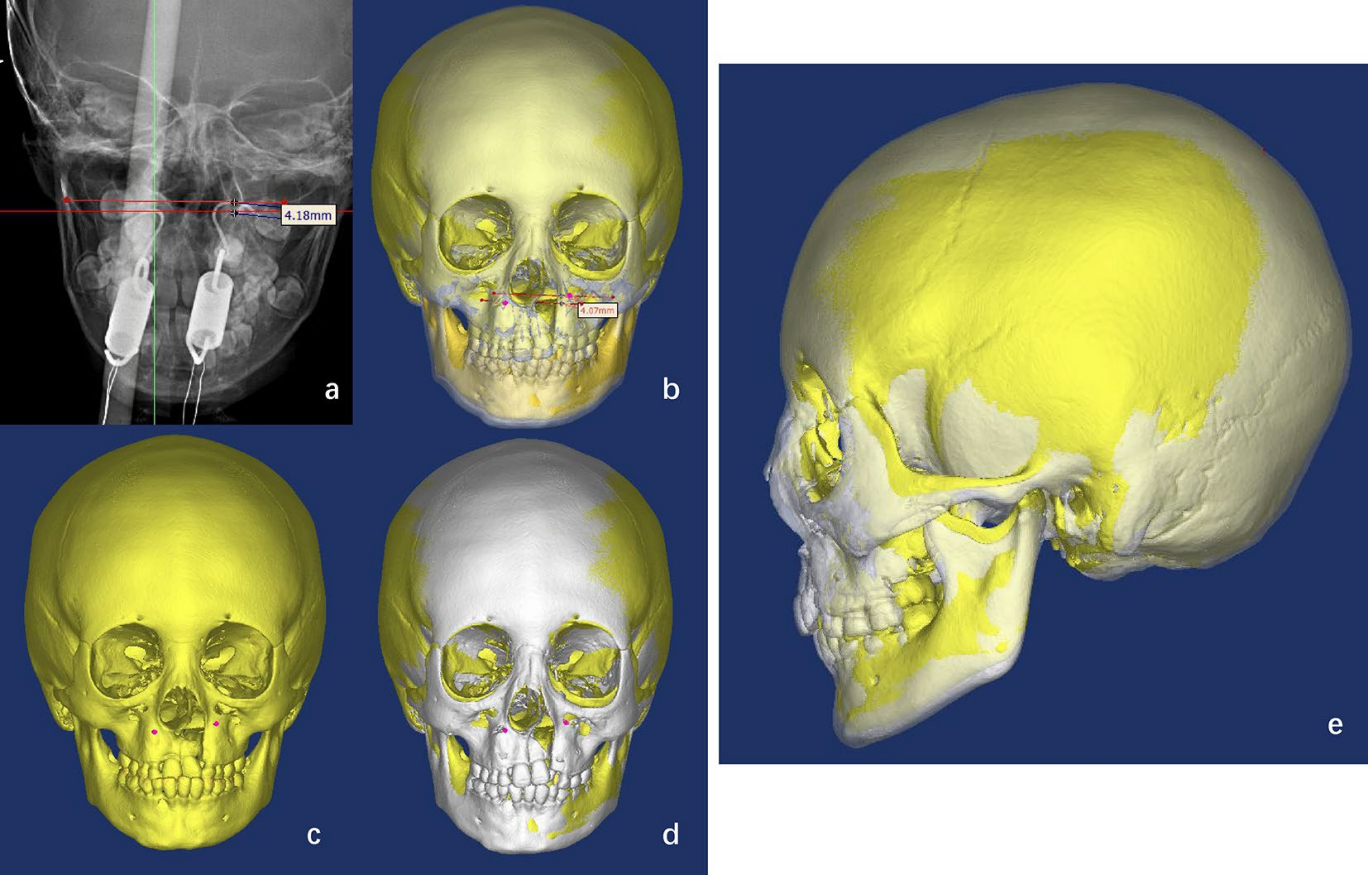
Evaluating the safety and effectiveness of the OSNS. (a) A cranial X-ray was performed to clarify the position of the traction hook; (b–d) The preoperative 3D reconstructed image was used as the base coordinate system, and the 3D cranial model was reconstructed after treatment (after removal of the traction hook), fitted to the base coordinate system to measure the changes in the morphological position of the patient’s maxilla; (e) The morphological position of the patient’s maxilla before and after treatment.
